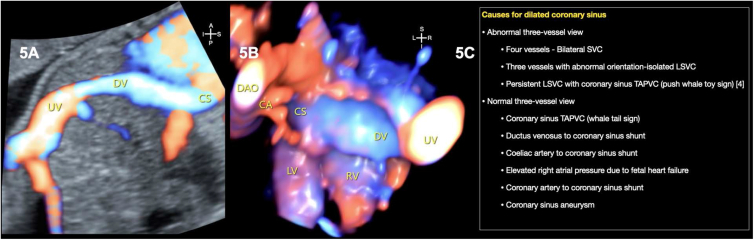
2-and 3-dimensional Fetal Echocardiography of Ductus Venous to Coronary Sinus shunt in a 34 Weeks Fetus and the Various Causes for Dilated Coronary sinus in Fetus (A and B) DV to CS shunt. (A) Power Doppler sagittal view of the fetal upper abdomen and lower thorax showing anomalous drainage of DV into the CS. (B) STIC power Doppler posterior 4-chamber view showing the anomalous drainage of DV into CS. CA = celiac axis; DV = ductus venosus; other abbreviations as in Figure 1, Figure 2, Figure 3, Figure 4.
